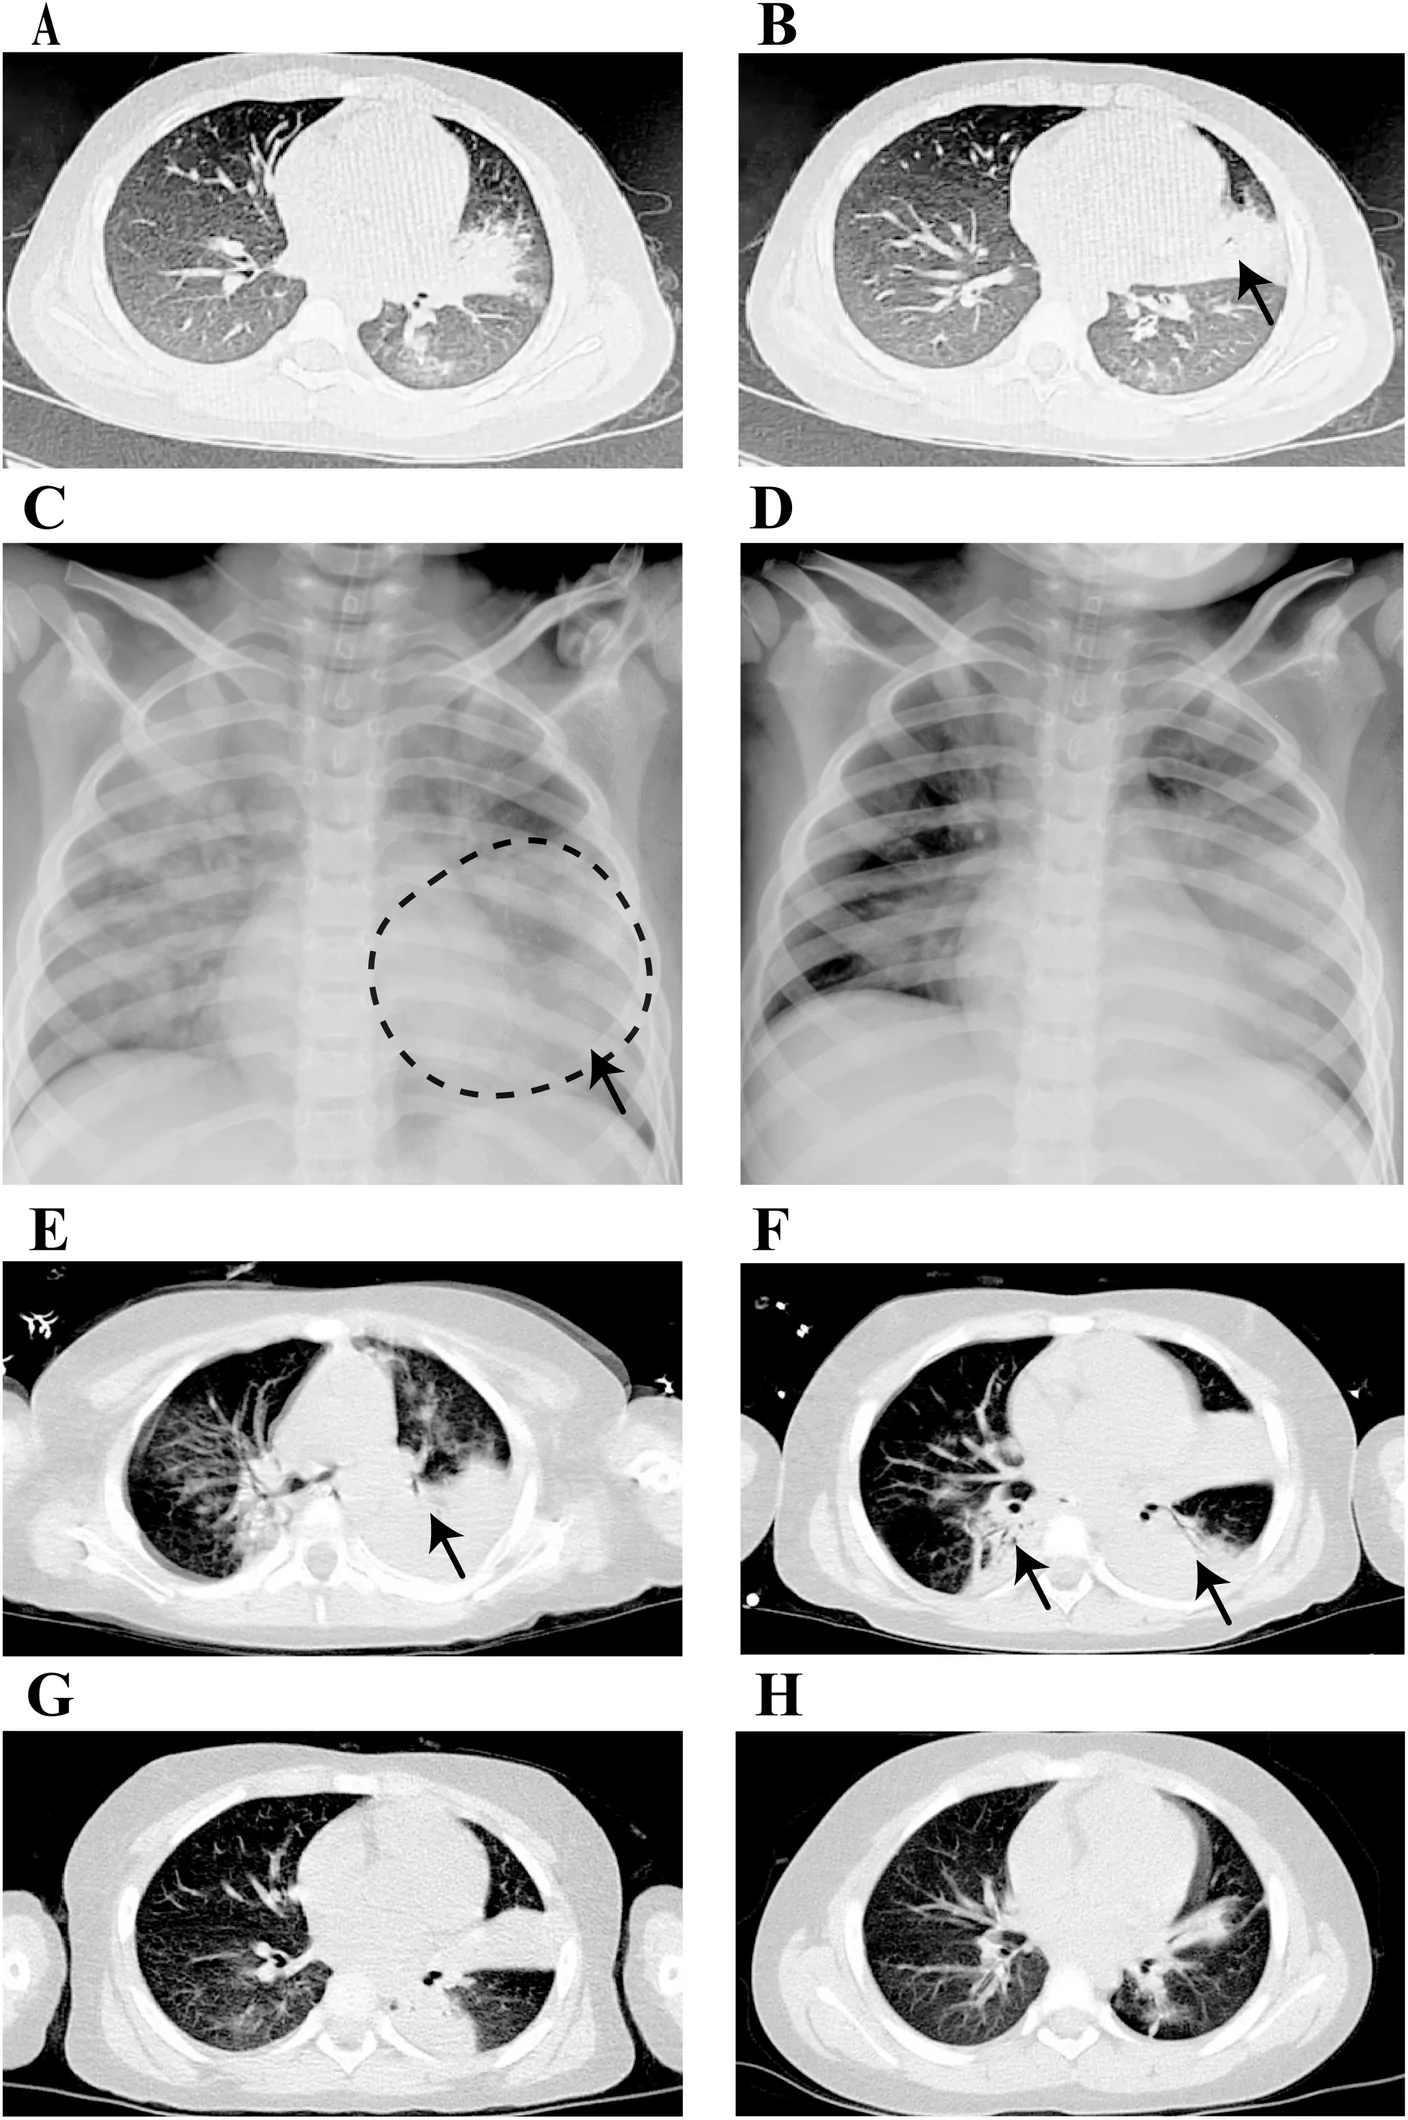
(A,B) chest CT before the first bronchoscopy showed inflammation and high-density opacities in the left lung, with a small amount of air bronchograms visible internally (arrows). (C) Immediate postoperative chest imaging revealed bilateral diffuse exudative opacities with left lung consolidation (arrows). (D) On postoperative day 3, the exudative opacities showed significant resolution. (E,F) Chest CT on day 6 after transfer demonstrated left upper lobe consolidation involving more than two-thirds of the lobe with distal bronchial obstruction, as well as partial consolidation in both lower lobes with air bronchograms (arrows). (G) Pre-discharge imaging revealed only focal consolidation in the left lung. (H) Follow-up CT showed near-complete resolution of the consolidative opacities.
